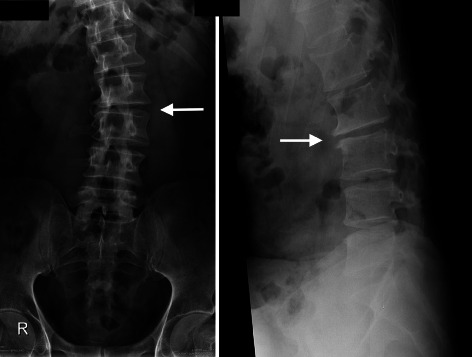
Lumbar spine radiographs show degenerative scoliosis with the apex at L2-3 (arrows).
